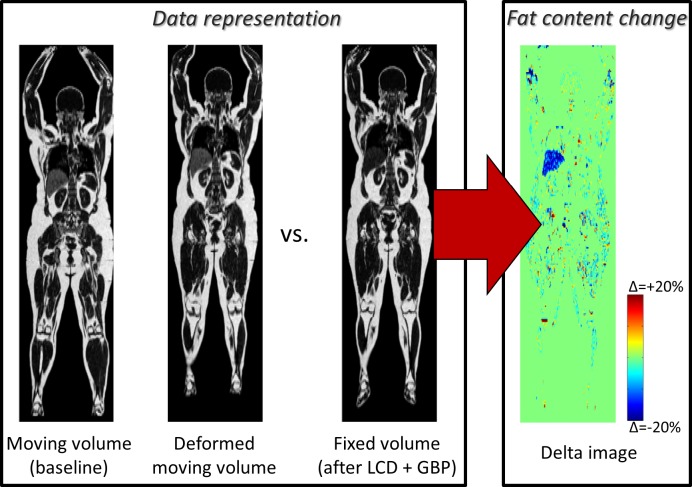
Longitudinal analysis. One coronal slice of the moving, deformed moving and fixed images of absolute fat content is shown together with a difference image between the fixed and deformed moving images. The subject underwent low calorie diet (LCD) and gastric bypass (GBP) between the moving and fixed MRI-scans. The total weight loss was 14 kg.
